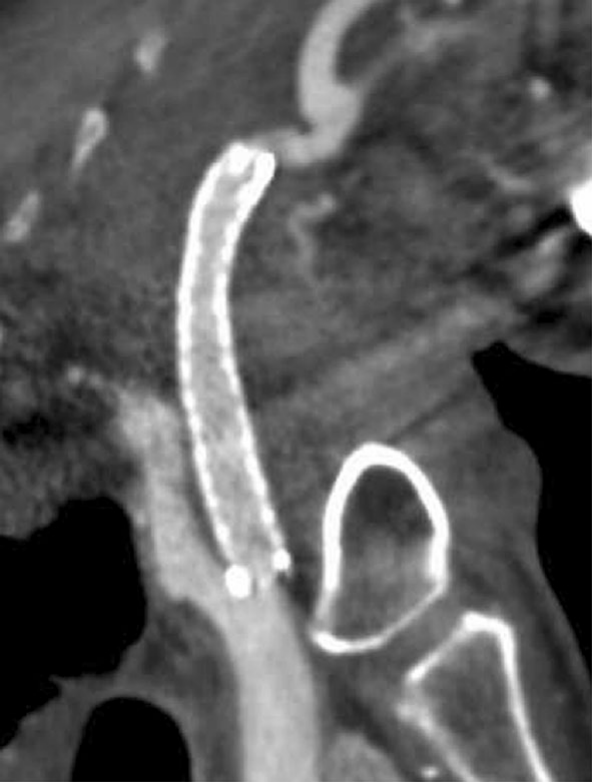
Multiplanar reconstruction contrast-enhanced CT in a sagittal view 2 months after stent placement shows full expansion of the stent and favorable patency of the carotid artery
